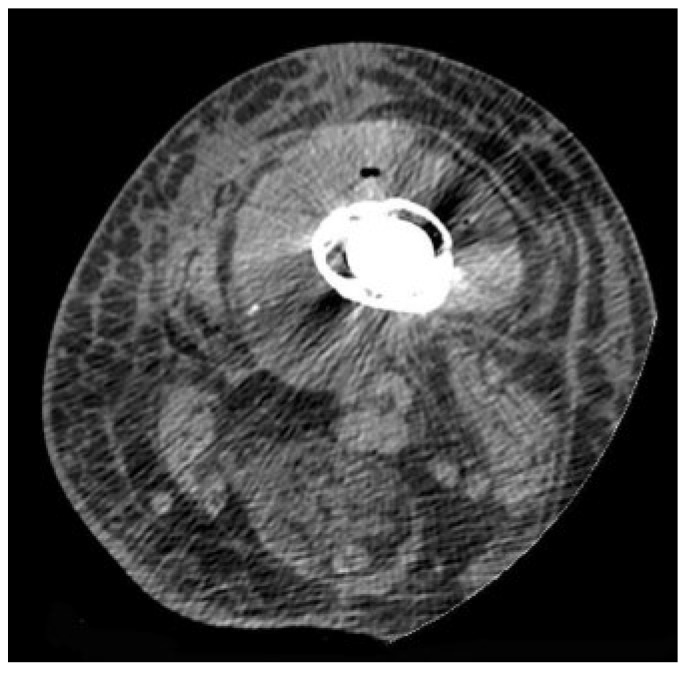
CT scans demonstrate fluid collection and increased density around diseased bone with a prosthetic implant, as well as swelling and hyperdensity of soft tissues due to edema [68].
